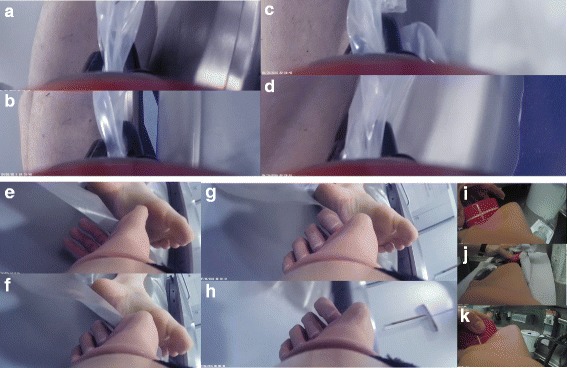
Socket mounted camera with video stills from workplace video documentation. CBPA (a-d) successfully grasps and holds on to plastic cover to pull body over from striker to CT table. TBI (e-h) can be seen to slip off not able to pull about 70 kg body weight by pulling plastic cover. Also, TBI fails to open jar with petrochemical substance (i-h) despite cleaning jar surface to make it less slippery
